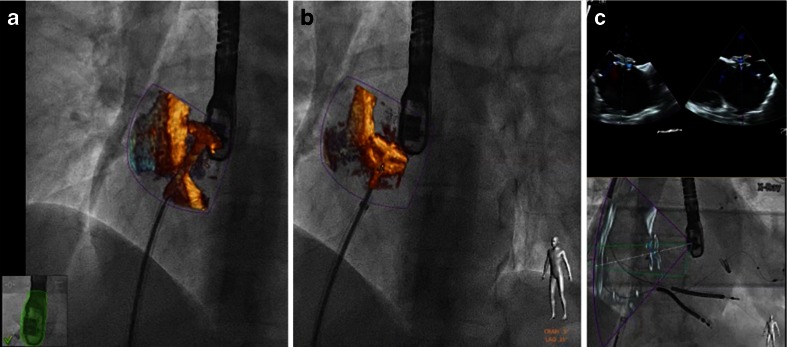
Fusion imaging guided ASD closure: 2D and 3D real-time TEE overlay on fluoroscopy. Using fusion imaging, the location of the sheet orifice is constantly visualized and the left atrial disc is released under direct vision (a). The right atrial disc is opened under echo-guidance (b). Color Doppler echocardiography overlay helps to determine correct development, localization, and function of the device without the use of contrast agent (c, different patient than a and b)
